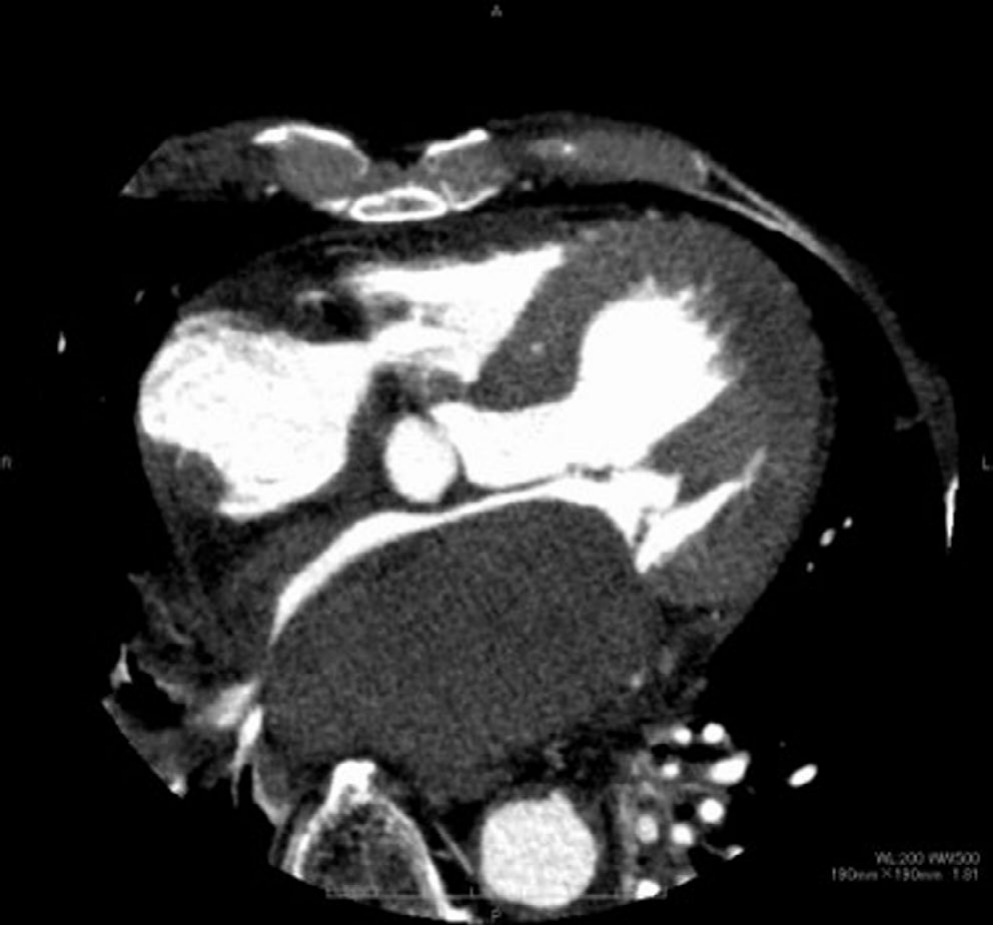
Preoperative computed tomography showing a large left atrial mass
